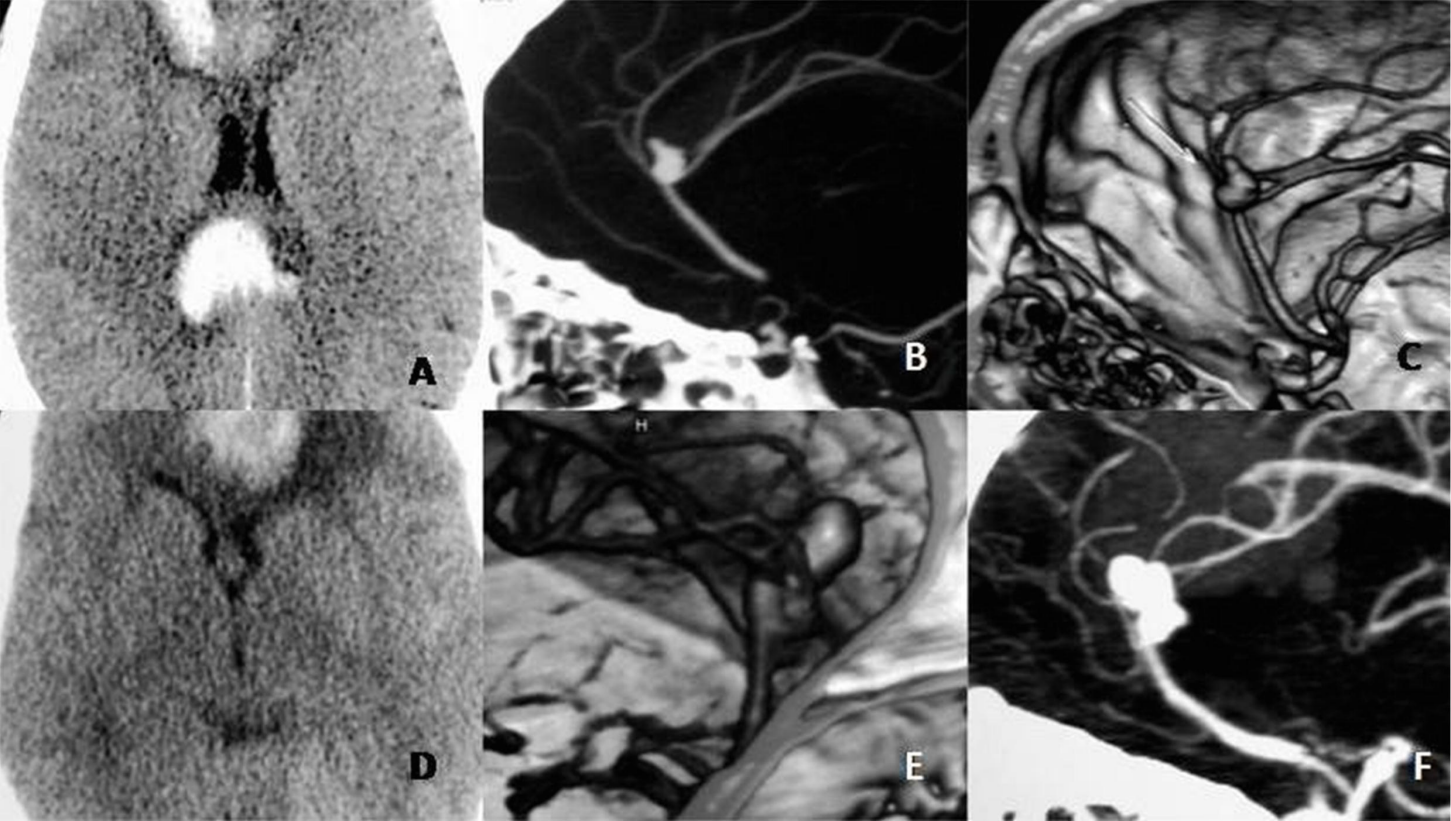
Preoperative Noncontrast CT and CT angiography images Images A-C (Case 1) - showing saccular aneurysm arising from an Azygos A2 ending in a quadrifurcation. Images D-F (Case 2) - showing Azygos A2 ending in a bifurcation, with close origins of distal branches that may give a pseudo-quadrifurcation pattern on angiography.
